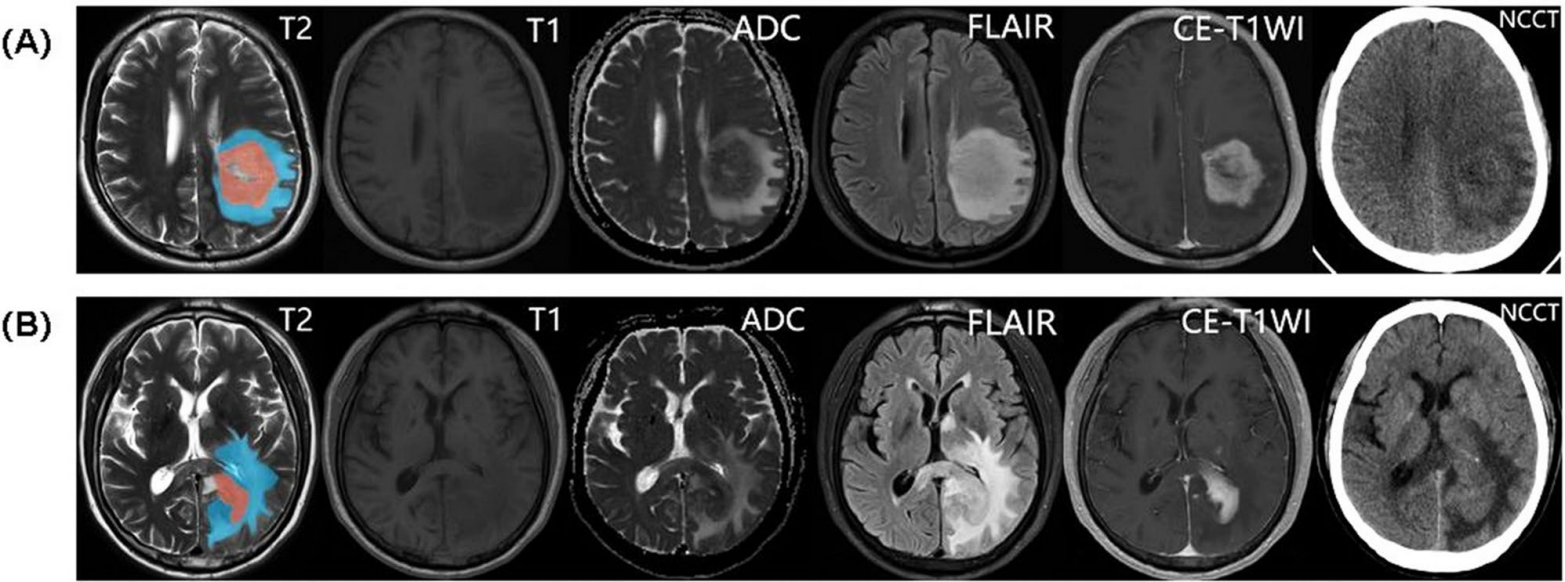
Multimodal imaging findings of representative cases of GBM (A) and PCNSL (B). From left to right: T2-weighted imaging (T2WI), T1-weighted imaging (T1WI), apparent diffusion coefficient (ADC) map, fluid-attenuated inversion recovery (FLAIR), contrast-enhanced T1-weighted imaging (CE-T1WI), and non-contrast computed tomography (NCCT). ROI masks are overlaid on the leftmost T2WI: CE region (red), and NE region (blue)
